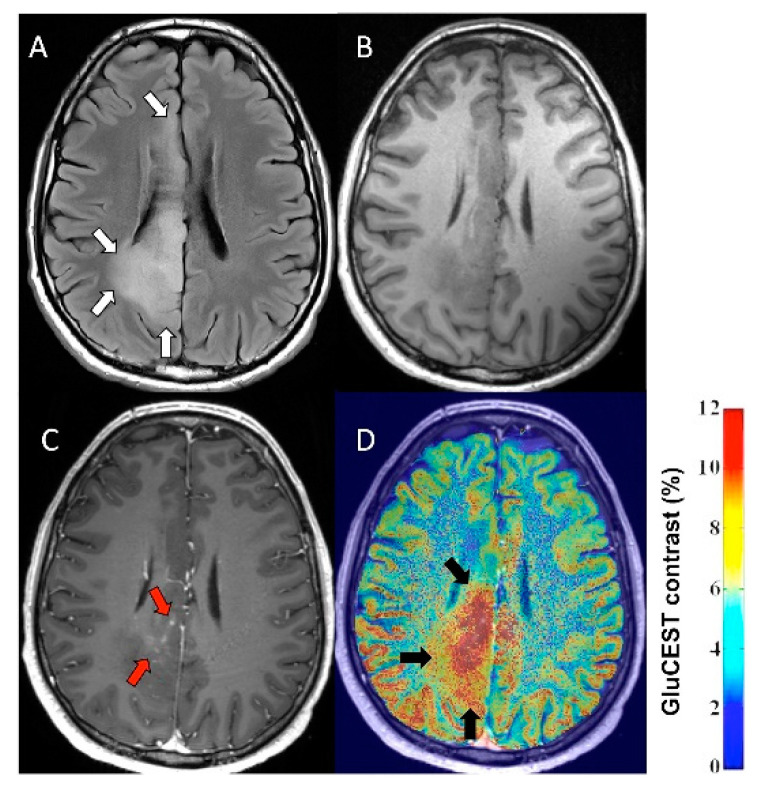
GluCEST contrast vs. gadolinium enhancement in a WHO grade II oligodendroglioma. (A) 3T FLAIR images in the axial GluCEST acquisition plane, tumor identified by white arrows. (B) 3T T1-weighted images without gadolinium contrast in the axial GluCEST acquisition plane. (C) 3T T1-weighted images with gadolinium contrast in the axial GluCEST acquisition plane with evidence of nodular, wispy mesial contrast enhancement (red arrows). (D) 3T T1-weighted images with gadolinium coregistered with GluCEST contrast maps. Increased GluCEST contrast in a region overlapping but extending beyond the area of gadolinium enhancement (black arrows). (Reproduced from Figure 2 of Neal et al. [54]).
